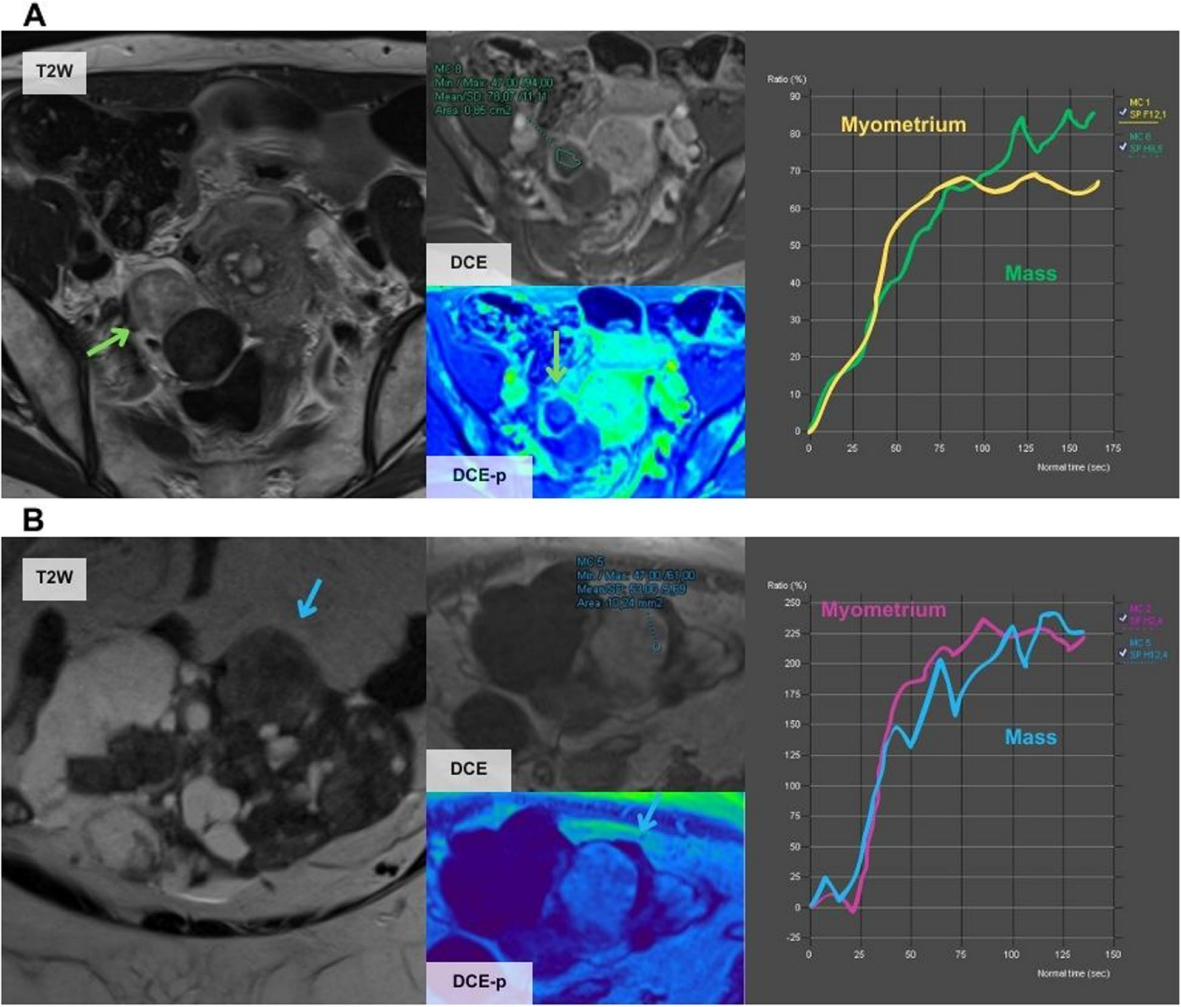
Erroneous classifications by the JR and the SR. A A 61-year-old woman presented an incidental right ovarian mass (green arrow) with a high-risk TIC classified as score 5 by both readers but was finally a fibrothecoma. As specified by O-RADS MRI guidelines, unenhanced sequences should be acquired before the contrast bolus injection. However, in this case, there was an error in the acquisition as there were no unenhanced sequences before the injection of the contrast bolus (in both TIC the contrast uptake started at the second 0), and this can distort the TIC results and lead to misinterpretation. In clinical practice, cases like this should be considered O-RADs 0 (incomplete or erroneous MRI technique). B A 56-year-old woman presented an incidental left ovarian mass (blue arrow) with a high-risk TIC that was classified as score 5 by both readers but was finally a luteinised fibrothecoma
